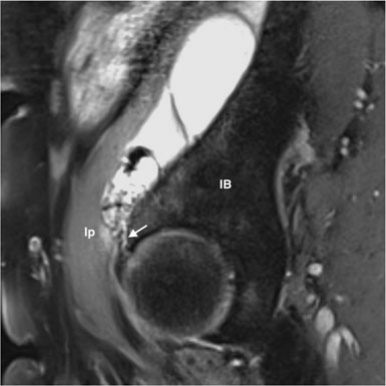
A giant synovial cyst incidentally found in the right hip of a 67-year-old woman during a routine computed tomography scan in the follow-up of a colorectal cancer in complete remission. For better characterization of the lesion, MRI was performed. Sagittal PD-WI shows a smooth, large multiloculated cyst, communicating with the joint space through a stalk (arrow). The lesion, probably corresponding to an enlarged iliopsoas bursa, displaces anteromedialy the iliopsoas muscle, and despite its close contact with the iliac bone, any erosion is seen. Ip, iliopsoas; IB, iliac bone
